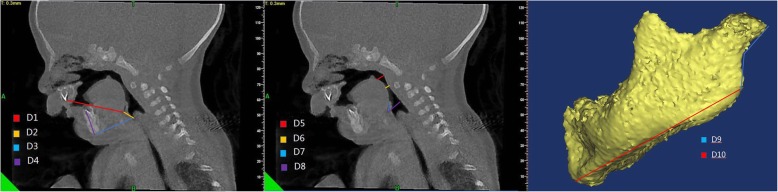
Upper airway distances D1–D11 derived from 3D reconstructions of craniofacial CBCT images acquired prior to mandibular distention osteogenesis for treatment of Robin sequence. Distances D1 to D10 are shown while D11 is the sum of D9 plus D10
